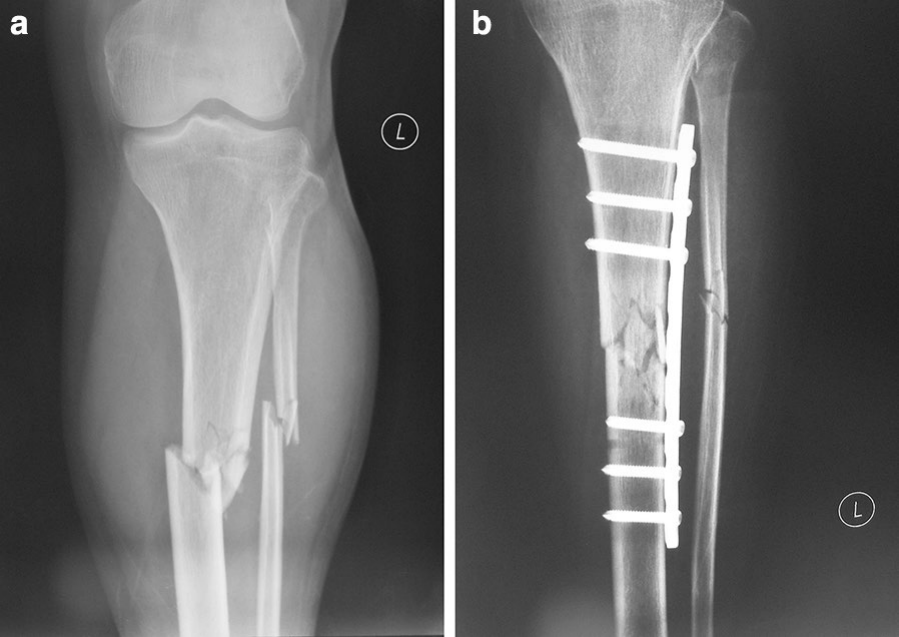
Preoperative radiograph (a) and radiograph immediately after incision reduction and internal fixation surgery (b) at the first visit to another hospital
